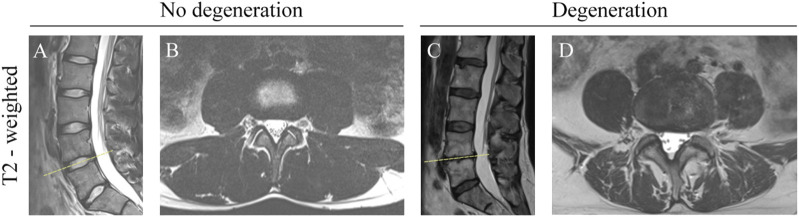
Morphometric Changes in the Lumbar Spine on T2-Weighted MRI. (A) Sagittal view: Non-degenerated discs showing hyperintense signal and normal height. (B) Transverse view: Non-degenerated facet joints with normal joint space and no osteophytes. (C) Sagittal view: Severe disc degeneration at L4/L5, indicated by hypointense signal and decreased disc height. (D) Transverse view: Facet joint degeneration at L4/L5, with obliterated joint space and osteophyte formation.
